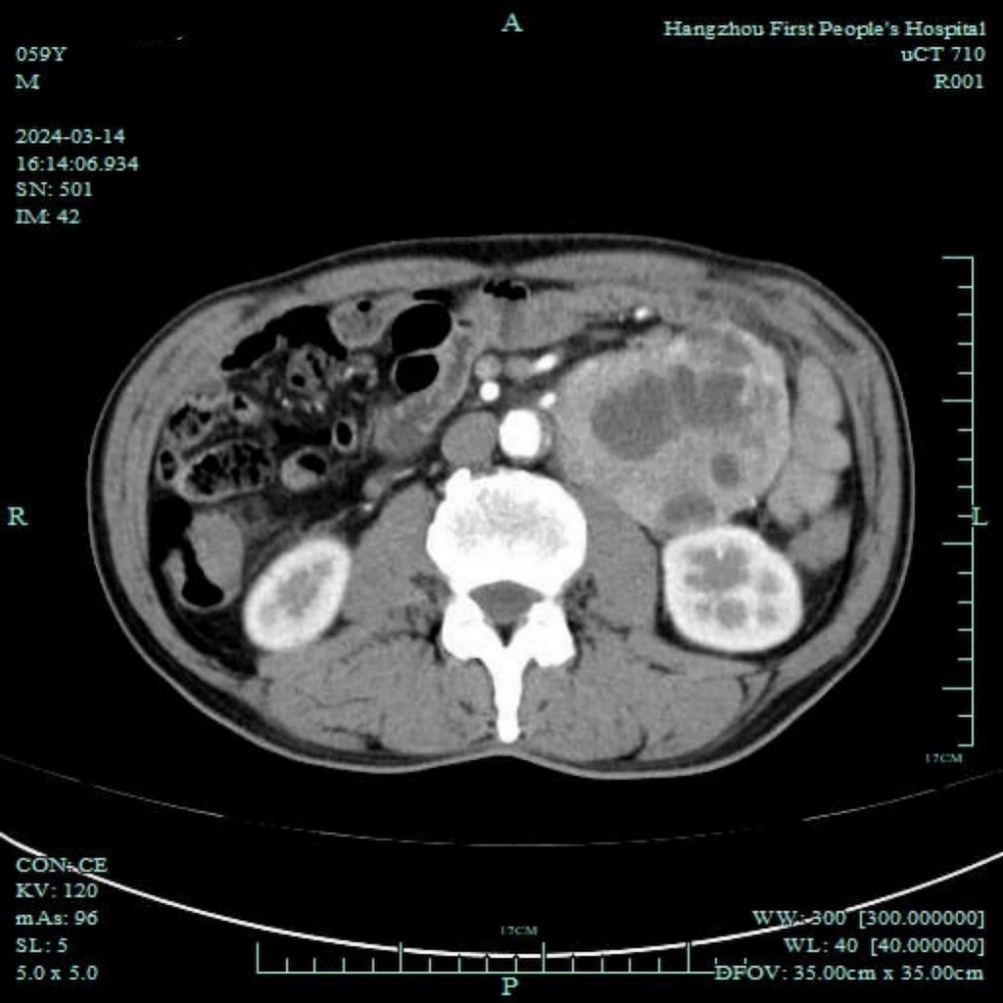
Abdominal enhanced computed tomography showing a lower-left abdominal tumor, approximately 91 × 70 mm in size, with indistinct boundaries adjacent to three segments of the small intestine, suggesting a malignant gastrointestinal stromal tumor or leiomyosarcoma.
